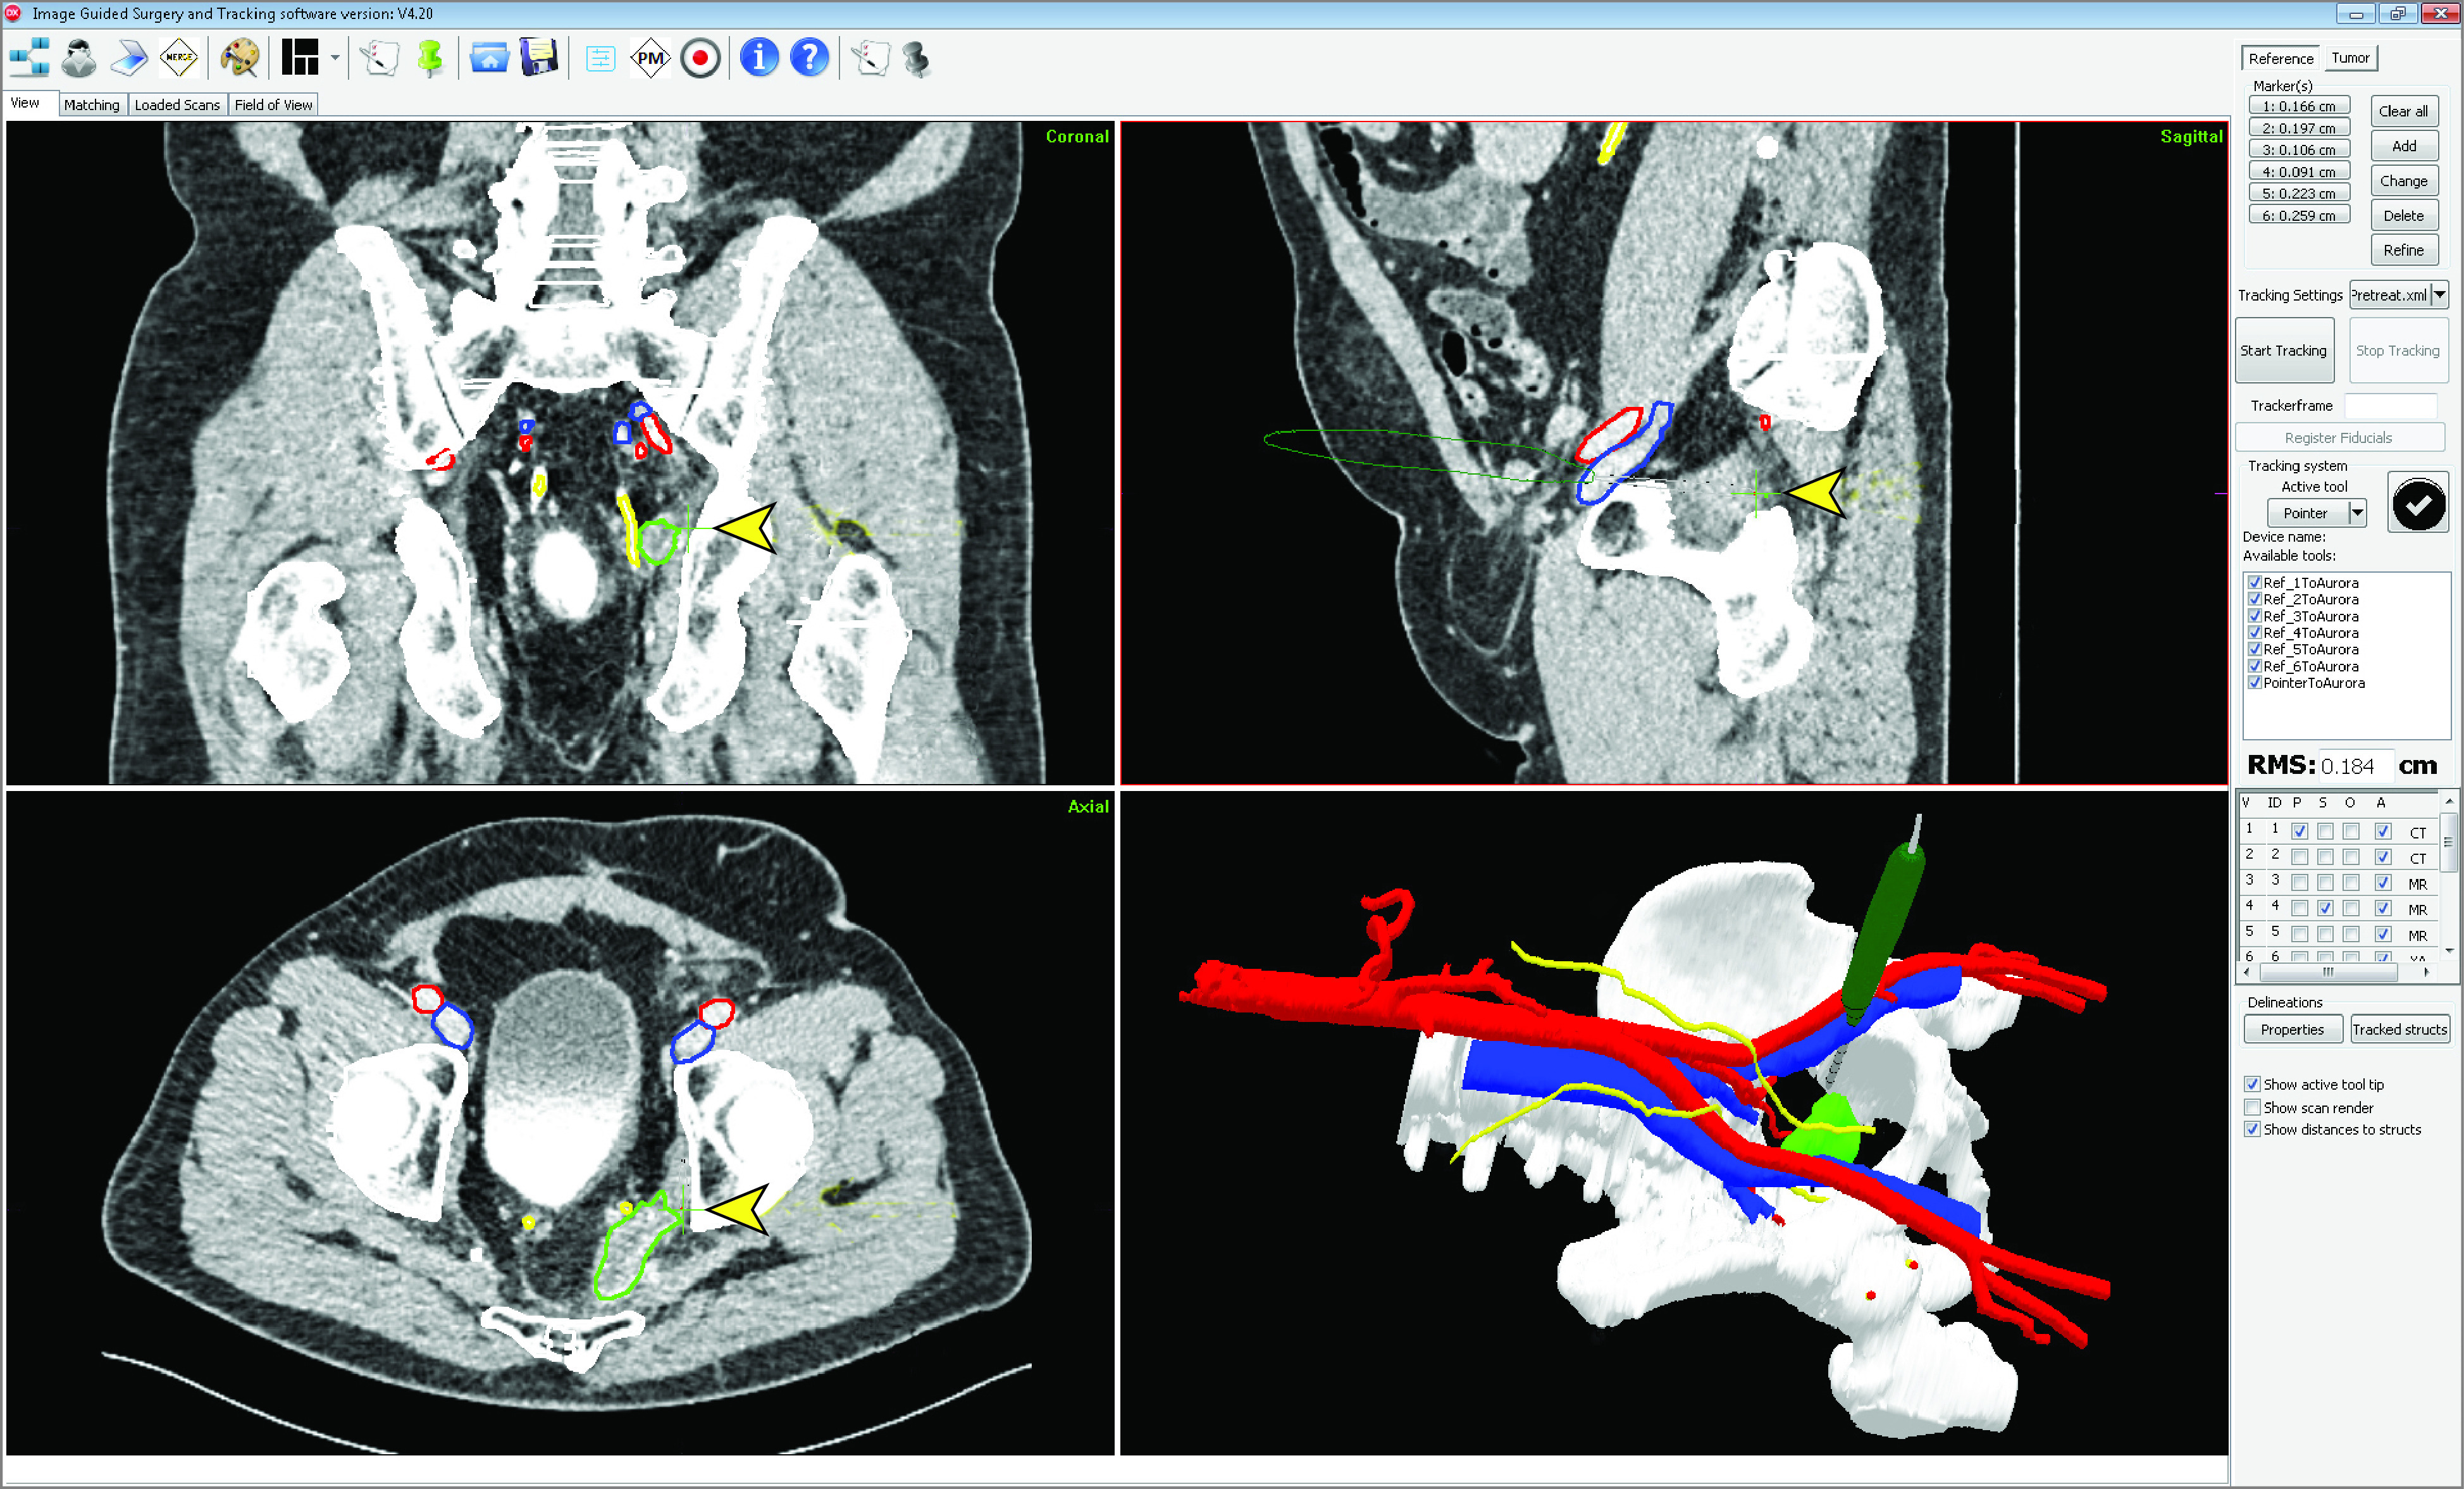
Example of the Image-Guided Navigation System Interface During Recurrent Rectal Cancer Resection The planning computed tomography scan, with segmentations in the coronal, sagittal, and axial planes, and the 3-dimensional model are shown. The surgical pointer is shown in the 3-dimensional model and orthogonal views (highlighted with arrowheads). The tumor is segmented in green, the ureters in yellow, and the vessels in blue/red.
